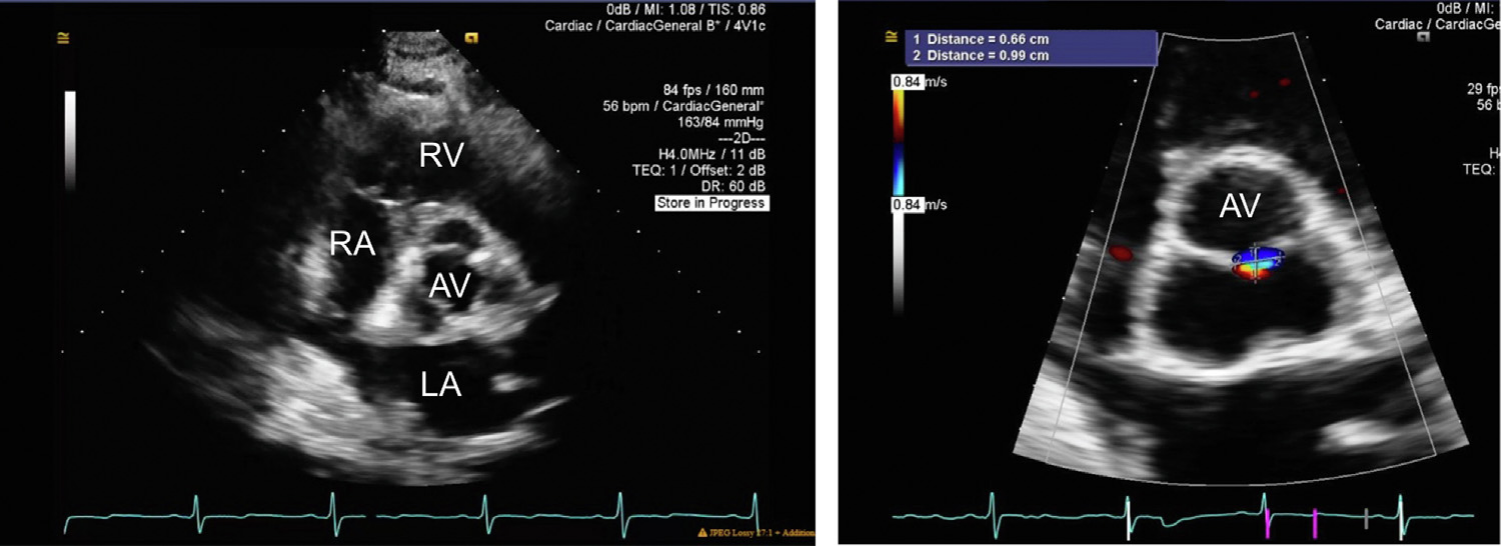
Two-dimensional TTE, parasternal short-axis view at the level of the aortic valve, diastolic phase, without (*left*) and zoomed with (*right*) color flow Doppler, demonstrates a tricuspid aortic valve with mild aortic regurgitation. *AV*, Aortic valve; *LA*, left atrium; *RA*, right atrium; *RV*, right ventricle.
